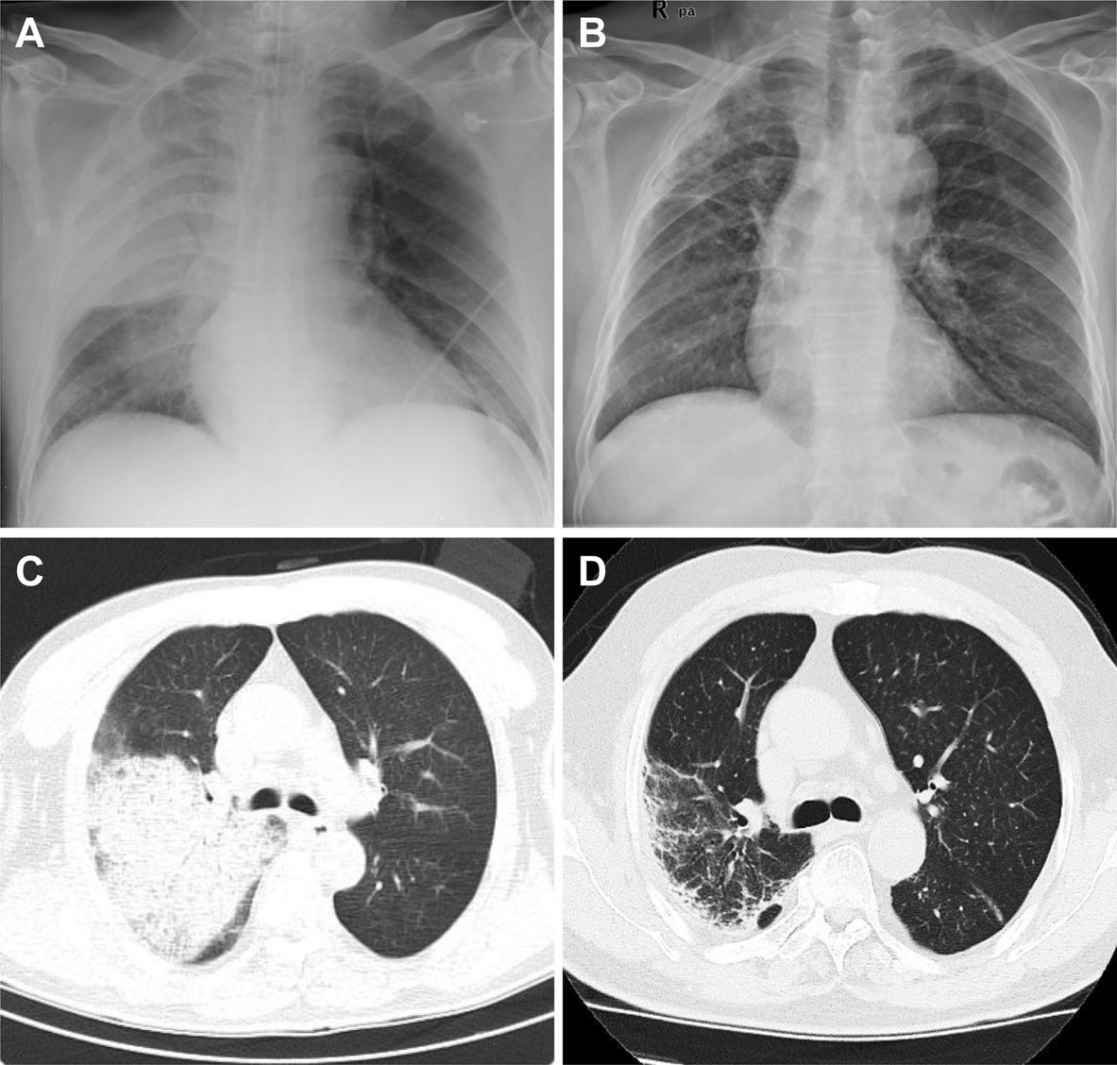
Chest X-ray and computed tomography on admission and the 28th day after admission. Chest X-ray on admission showed infiltrative shadow from the right upper, middle and lower lung lobes (a). Computed tomography on admission showed multiple exudate consolidation in the right upper, middle and lower lung lobes (c). On the 28th day after admission, the chest X-ray (b) and computed tomography (d) showed improvement in the consolidations of the right upper, middle and lower lung lobes
